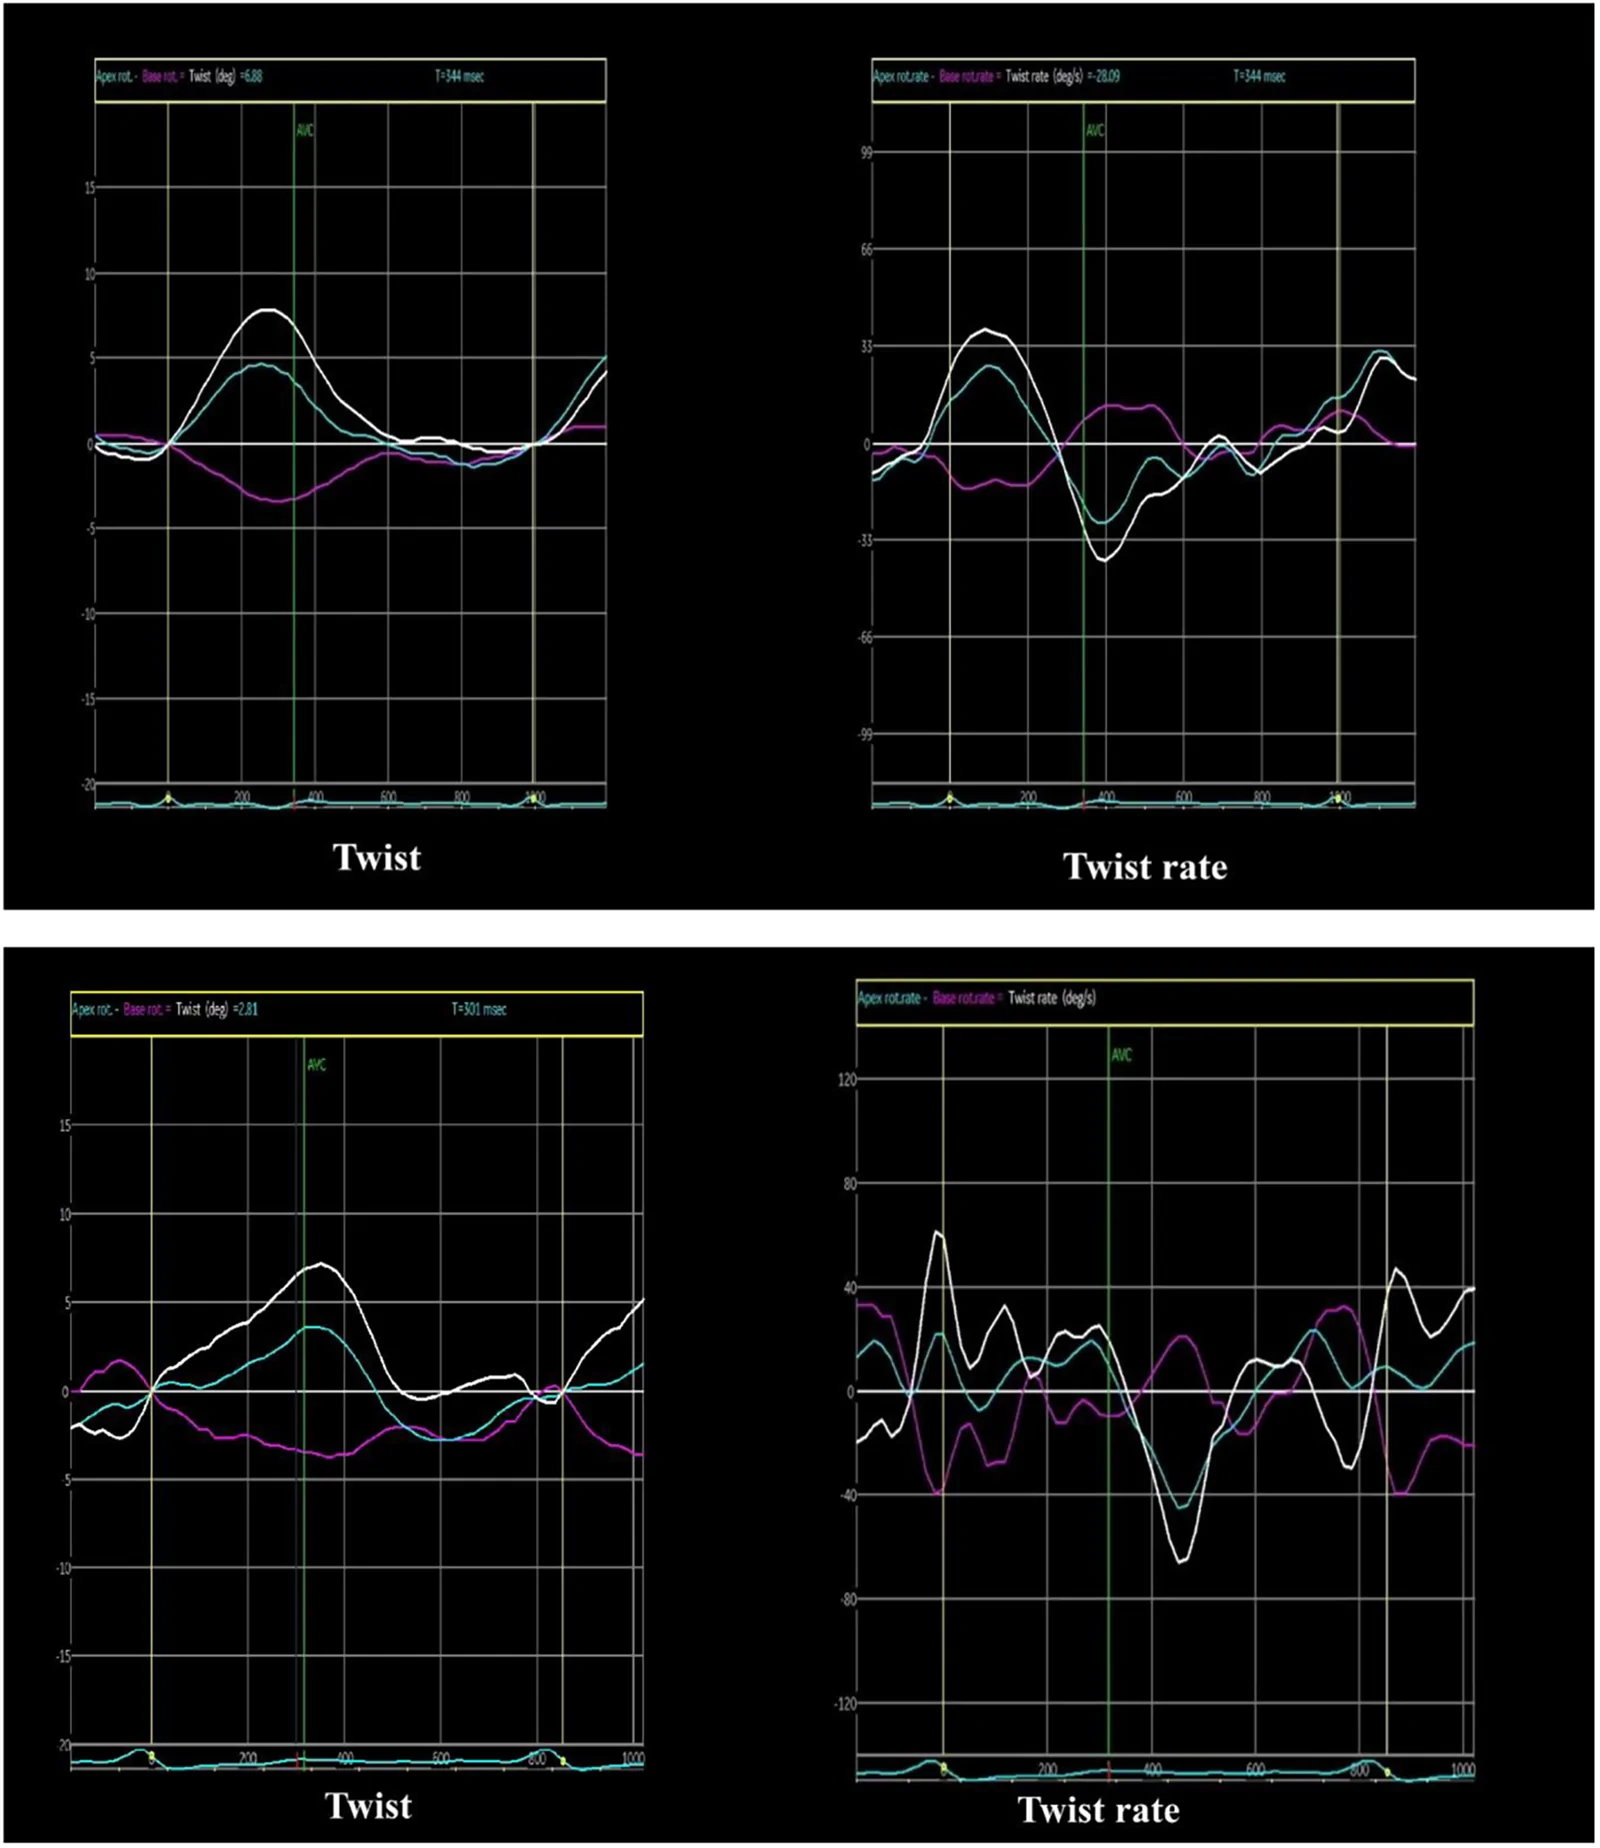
Torsional mechanics evaluated by 2D STE in normal condition (above) and in a patient with dilated cardiomyopathy and LBBB (below). In the normal heart (upper panels), LV twist increases smoothly and progressively during systole to a single, well-defined positive peak around aortic valve closure (AVC), and the twist-rate curve shows an orderly systolic peak followed by a coordinated diastolic untwisting (recoil). In the patient with dilated cardiomyopathy and LBBB (lower panels), peak systolic twist is markedly reduced and temporally delayed, and both the twist and twist-rate curves become irregular and multiphasic with loss of the coordinated systolic peak, reflecting impaired and dyssynchronous rotational mechanics. 2D STE, two-dimensional speckle-tracking echocardiography; AVC, aortic valve closure; LBBB, left bundle branch block; LV, left ventricle.
